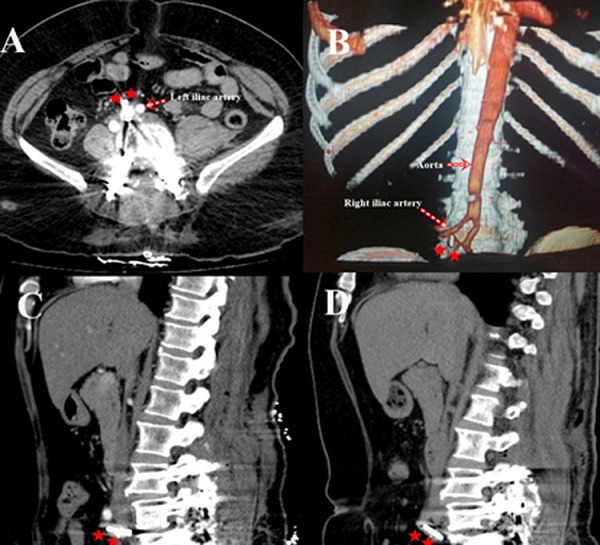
Axial (A) and sagittal (C) images of PLIFC() with antepulsion from the L5-S1 intervertebral disc space replaced nearby to the left iliac artery (B) is realized on the control CT performed postoperative first day. No movement change of the cage is seen in the postoperative 1 year control CT (D)
